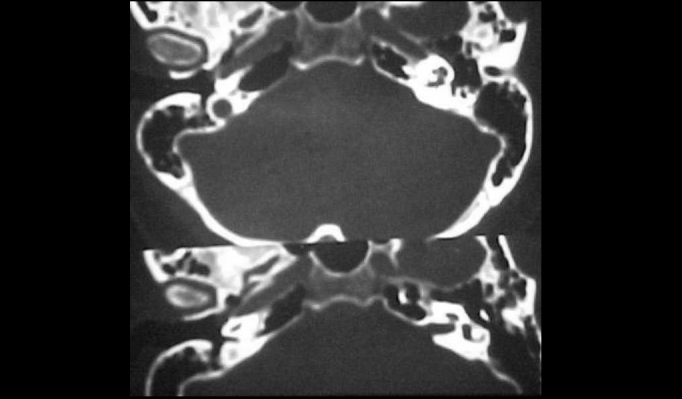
CT scan of temporal bone (axial section) showing circumscribed velamentum of the right promontory.
